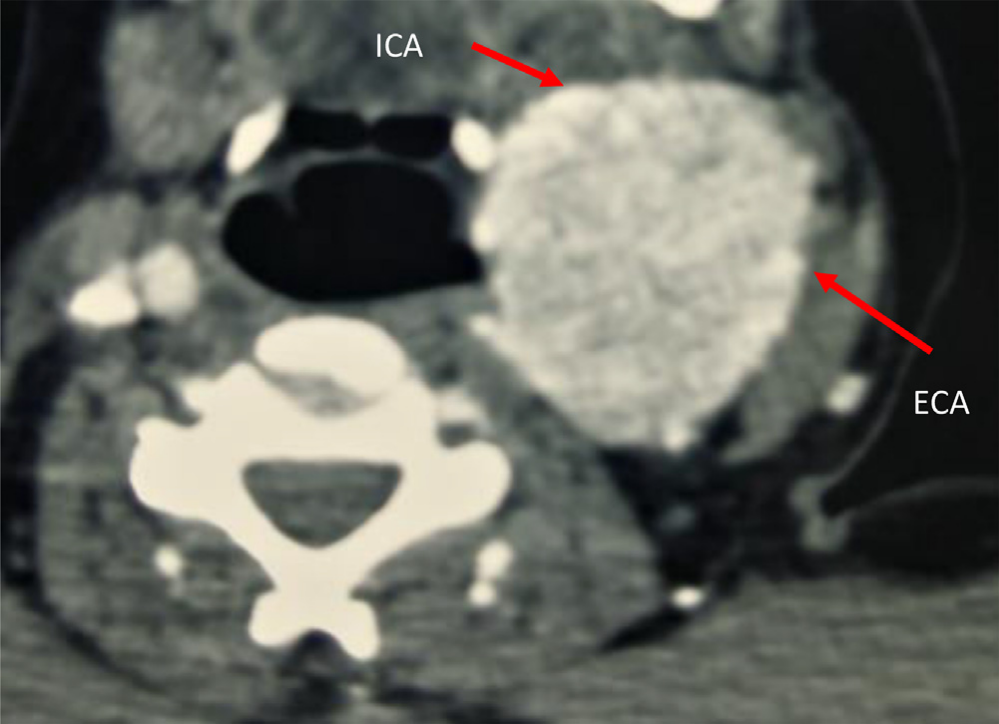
Axial CT scan (arterial phase) showing a large left cervical mass at the carotid bifurcation with intense enhancement. The internal carotid artery (ICA) and external carotid artery (ECA) are splayed apart (lyre sign).
